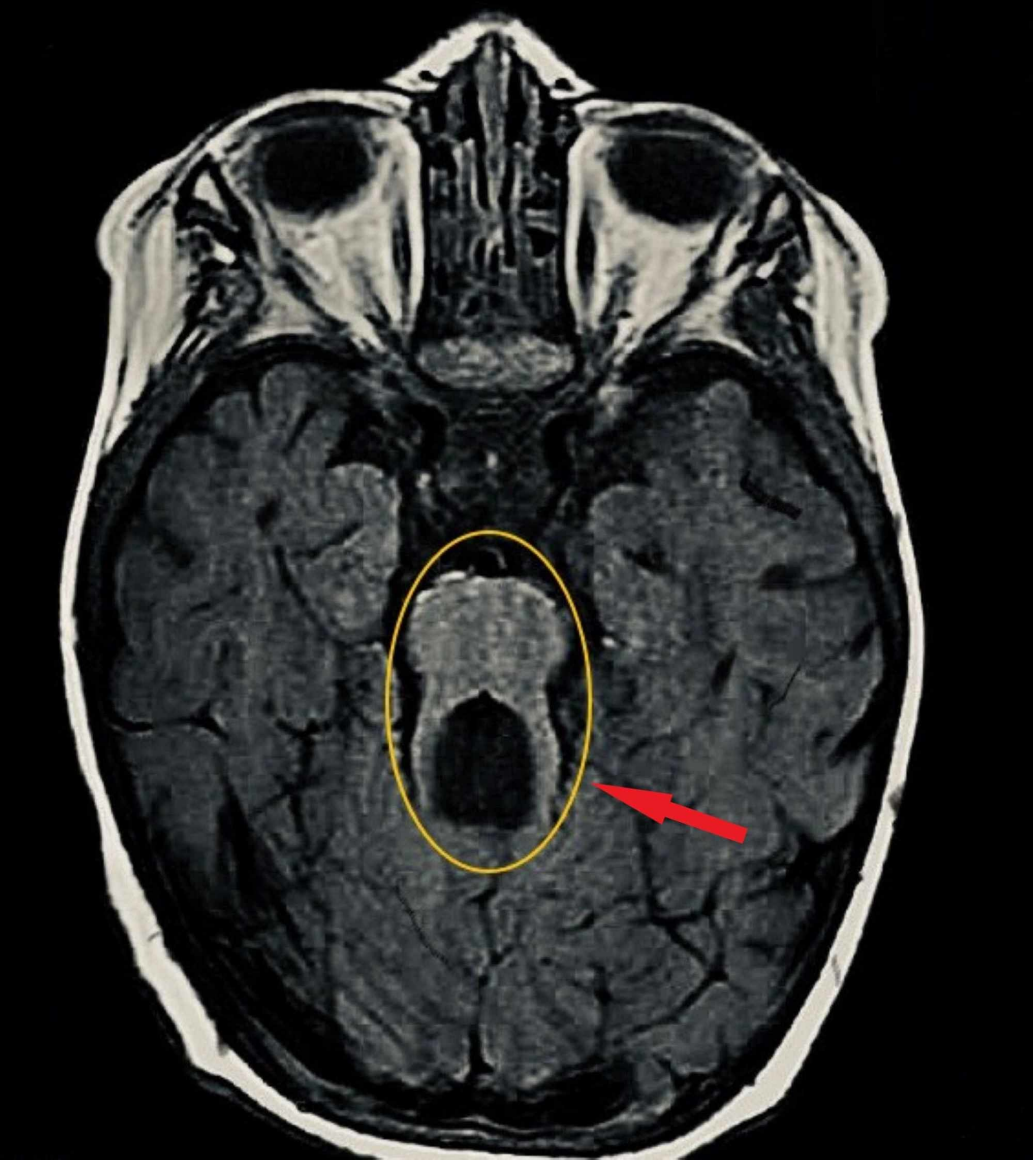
Classic sign of molar tooth seen in MRI of the brain
